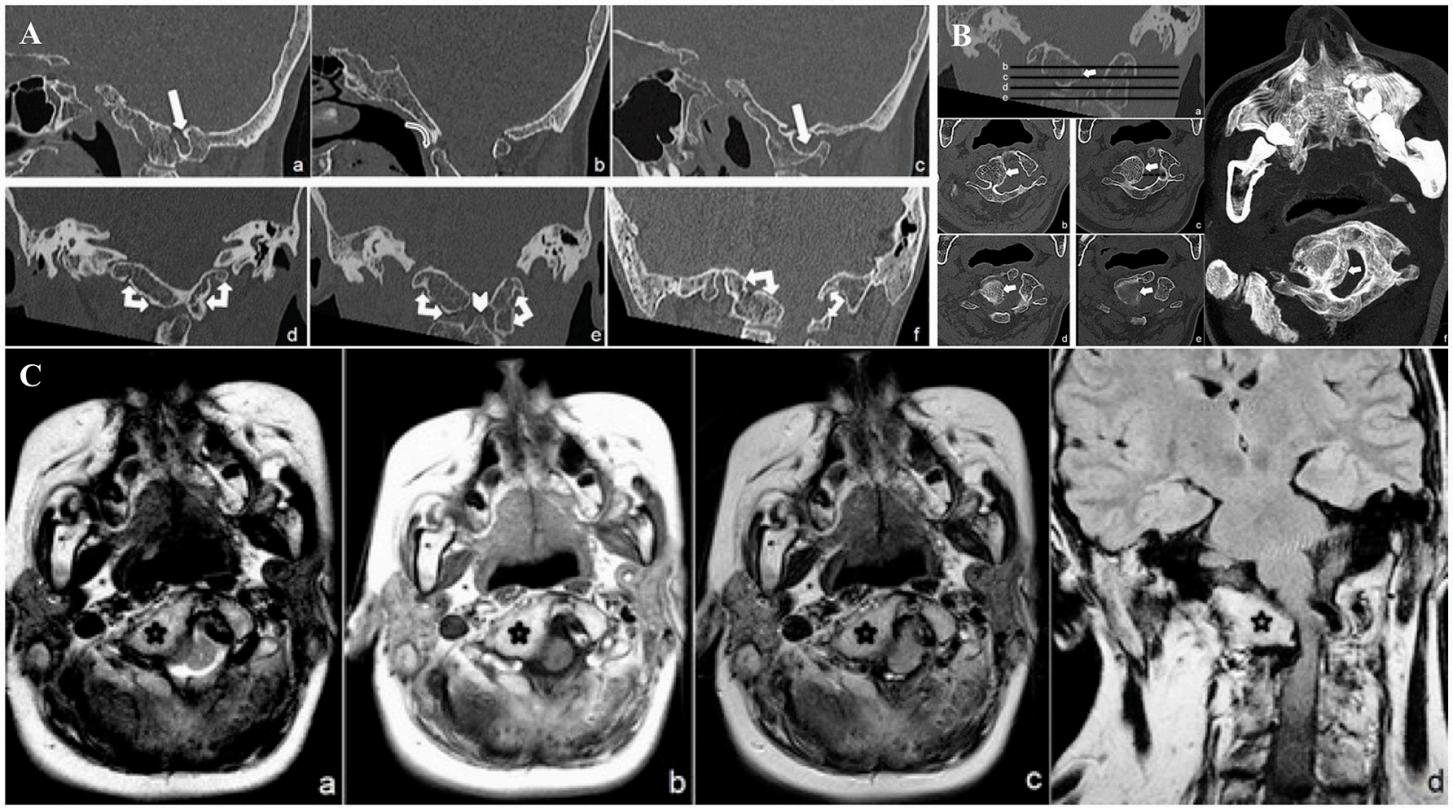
(A) CT minimum intensity projection (MIP) Sagittal (a: right parasagittal plane, b: midsagittal plane, c: left parasagittal plane) and Coronal (d: anterior atlanto-occipital complete fusion, e: lateral masses atlanto-occipital bilateral complete fusion, f: posterior atlanto-occipital partial fusion) CCJ reconstruction. Complete C 1 anterior arch and lateral masses fusion and partial fusion of the posterior arch to the occipital foramen (double-headed arrows). Foramen arcuate (white harrows). Note the “comma” appearance of complete anterior atlanto-occipital fusion (curved harrow) and the smaller odontoid process (arrowhead). (B) CT Coronal (a) and transverse minimum intensity projection (MIP) (b–e) and transverse shaded surface display (SSD) (f) reconstructions of narrowed foramen magnum due to right occipito-condylar hyperplasia (withe arrows). (C) MR transverse T2 (a), T1 (b), FLAIR (c) and coronal FLAIR (d) weighed sequences, show right ventrolateral compression of the lower medulla and upper cervical spinal cord (white arrow) at the level of narrowed foramen magnum due to CCJ complex malformation (black star).
